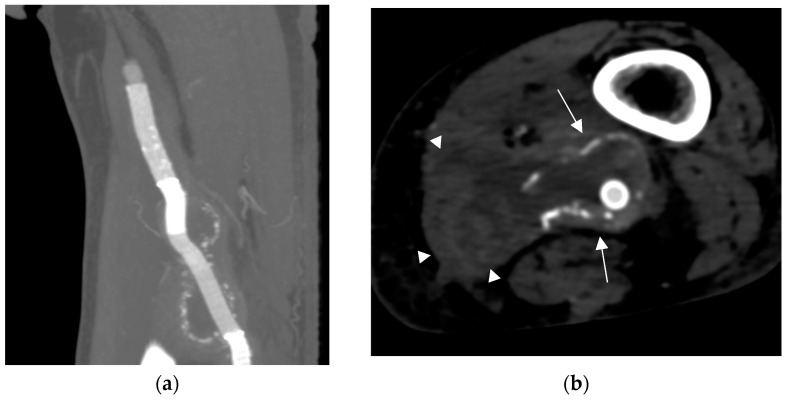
(a) CTA images showing the popliteal collection surrounding the popliteal stent graft; (b) CTA images showing the popliteal endograft (arrows) and the ruptured collection in the femoral muscles (arrowheads).
